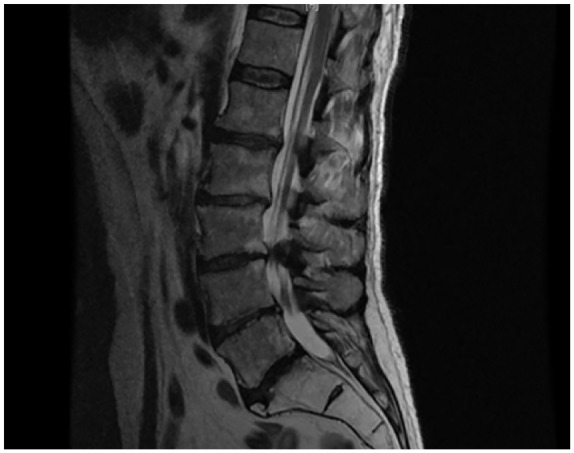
Repeat magnetic resonance imaging results on hospital day 2 revealed abnormal
